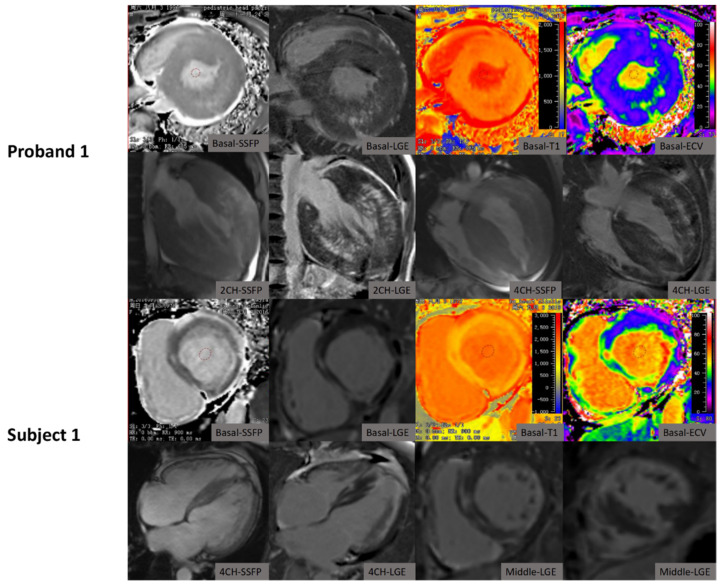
The characteristics of cardiac MRI in the proband 1 patient and the patient’s mother (Subject 1) with Danon disease.
